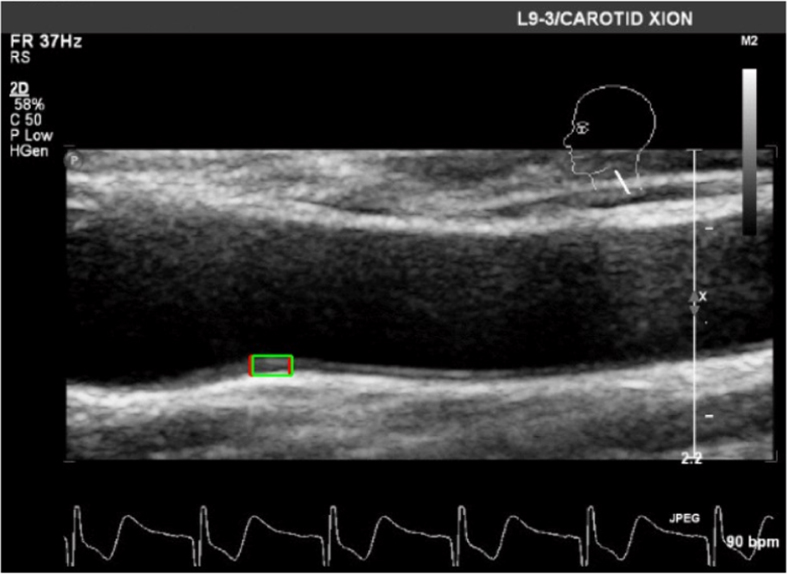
Image of the carotid artery wall displacement estimated from the b-mode ultrasound using region tracking and block matching.
